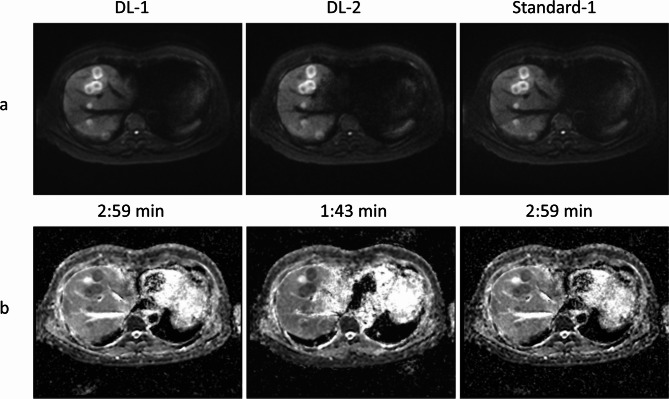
Example of b = 750 s/mm^2^ images (a) and ADC maps (b) from a 78-year-old male participant demonstrating multiple liver metastases. Contrast display per all b750 images and per ADC maps was kept identical. ADC = apparent diffusion coefficient; DL-1 = moderately-accelerated DWI using a deep-learning reconstruction algorithm; DL-2 = highly-accelerated DWI using a deep-learning reconstruction algorithm; Standard-1 = moderately-accelerated DWI using a standard reconstruction algorithm
